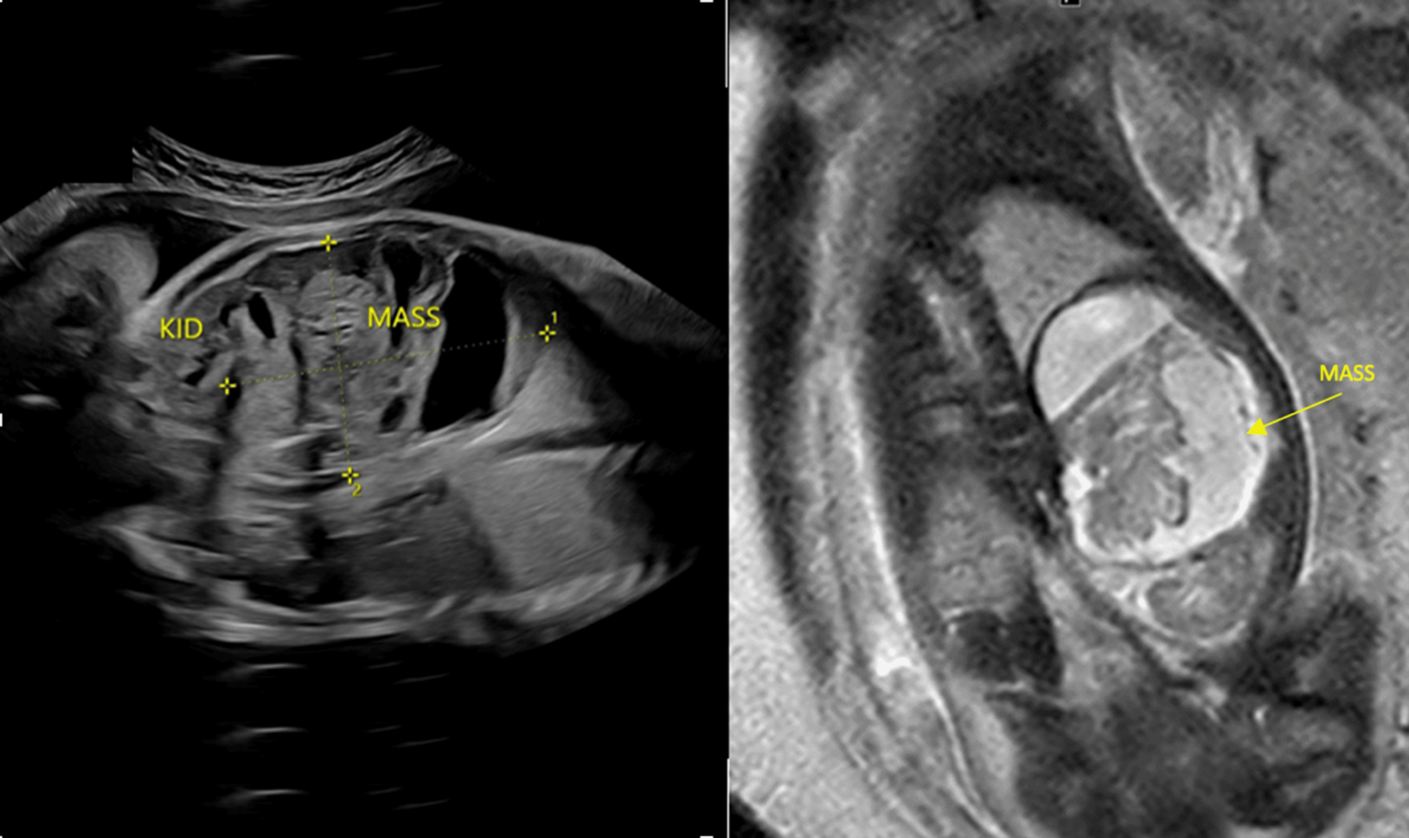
Fetal ultrasound (left) and fetal MRI (right) showing a large left suprarenal multi-cystic and solid lesion.
